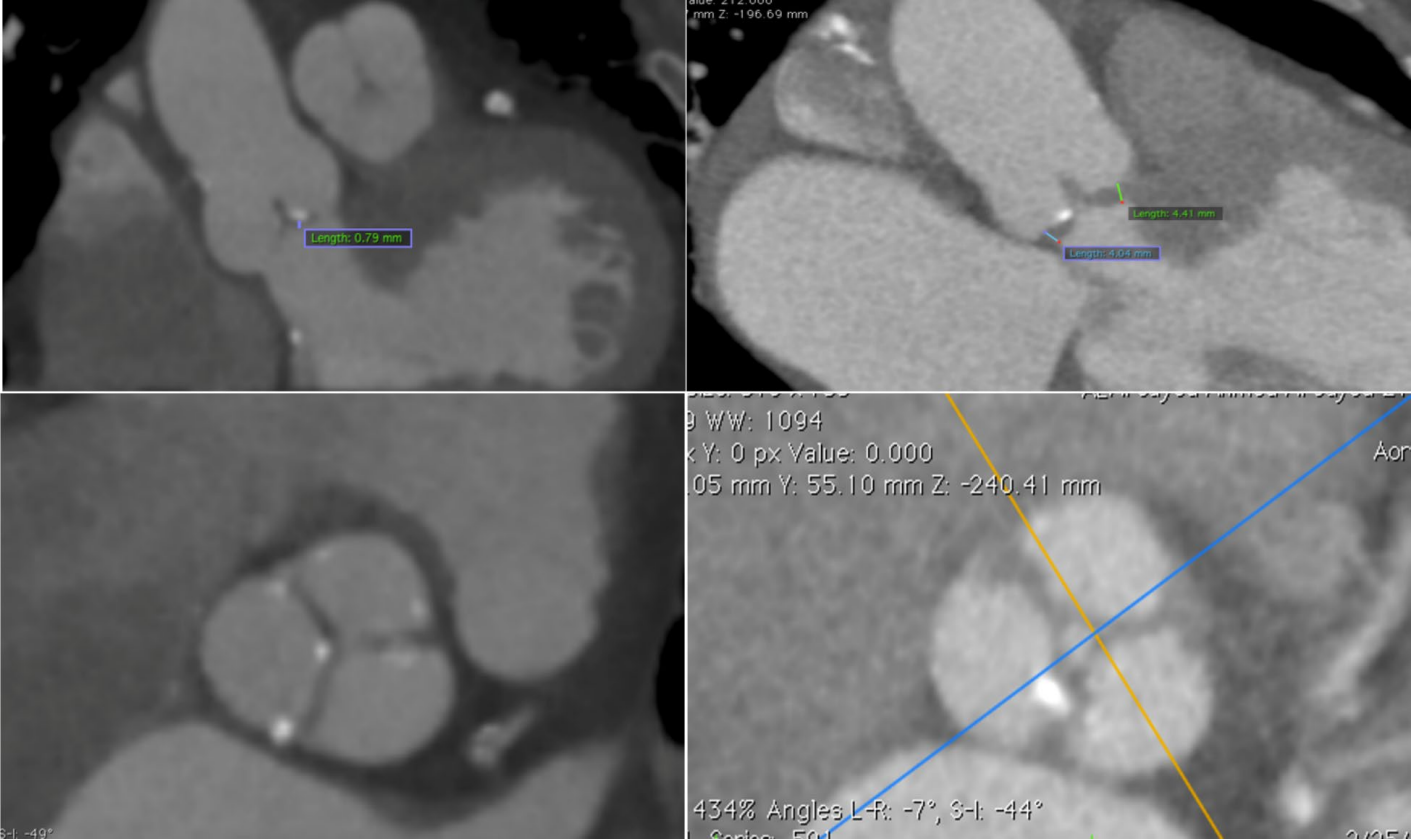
Pre-TAVI CT assessment of rheumatic severe AS
